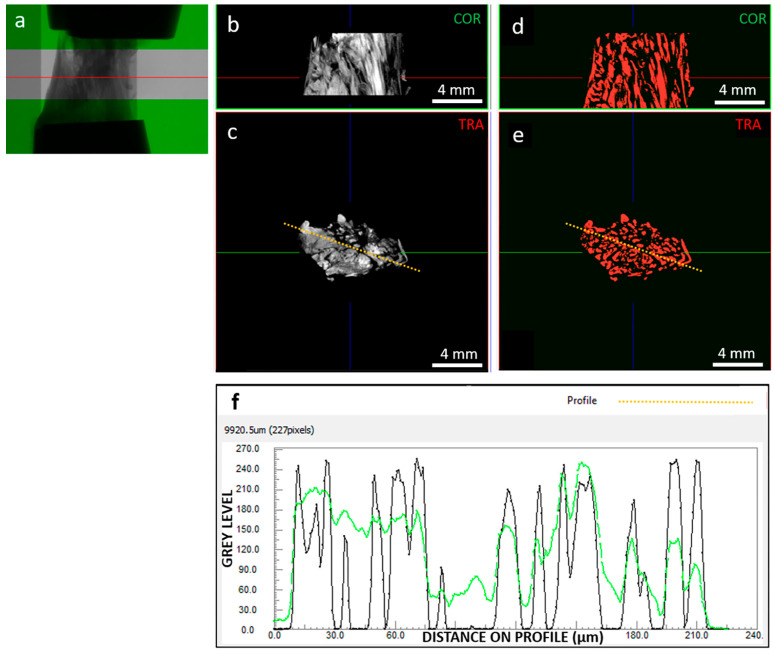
As an example, (a) radiography of clamped sample “A” at 0% strain highlighting out-of-green ROI; (b) coronal and (c) transversal sections of the grey level reconstructed image dataset, with corresponding coronal (d) and transversal (e) segmented dataset; (f) contrast profiles, in green for the orange dotted line on the grey scale image (c) and in black for the orange dotted line on the segmented image (e). Segmentation was able to resolve signal peaks corresponding to collagen fibers; in the example section, segmented objects (i.e., fibers) show an average thickness of 111 µm. The red line in images (a,b,d) indicates the transversal plane; the green line in images (c,e) indicates the coronal plane; the blue line in images (b–e) indicates the sagittal plane; the orange-dotted line in images (c,d) indicates the path along which the image contrast is profiled (f).
